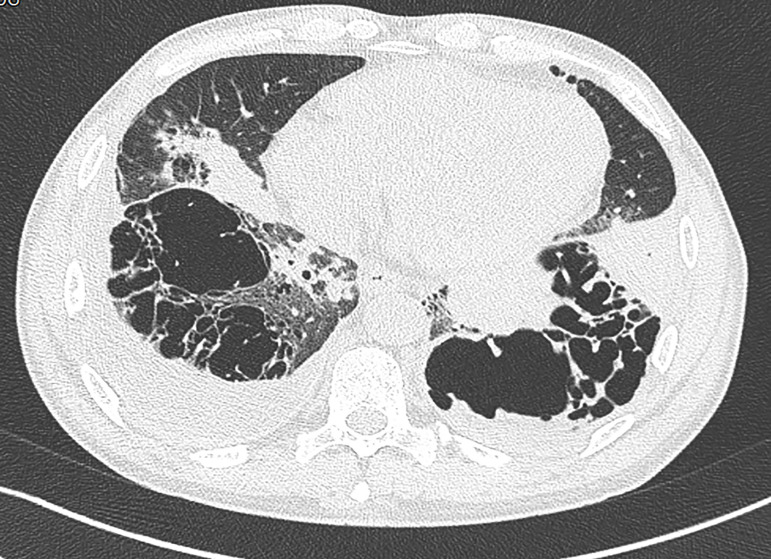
Chest computed tomography (CT): lung cavities with destruction of the lung parenchyma in the inferior lobes on both sides and a right pleural effusion.
